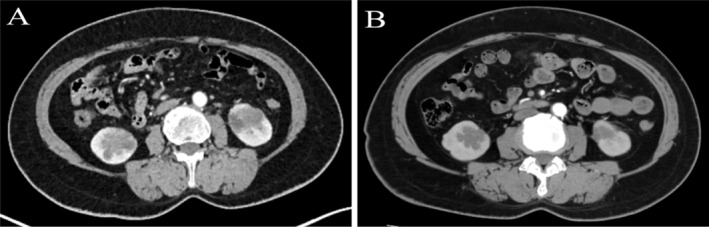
The enhanced CT scan of renal metastases. (A) Before targeted therapy; (B) 6 weeks after targeted therapy. Tumor response was judged as partial response.
